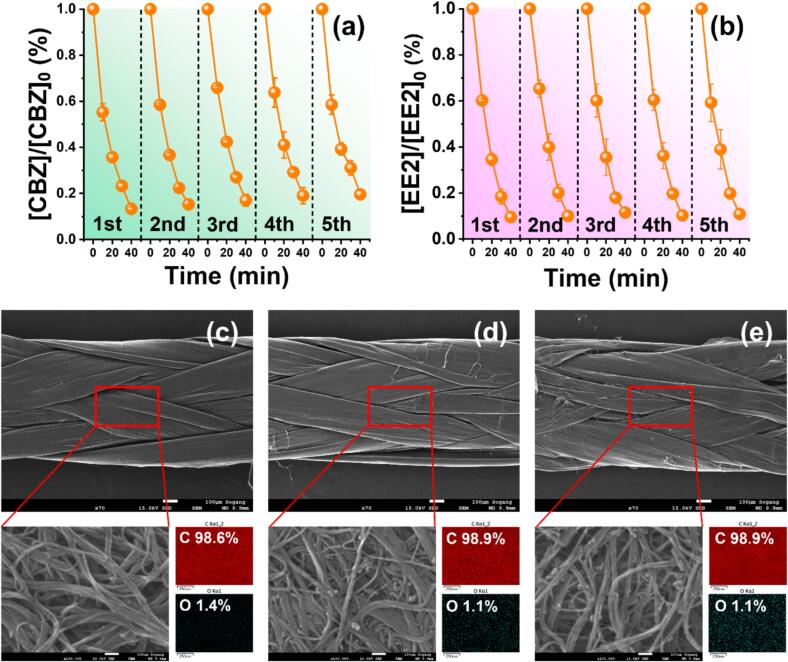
Reusability of CNTy over five consecutive treatment cycles for (a) CBZ and (b) EE2 ([CBZ]_0_ = [EE2]_0_ = 10.0 μM, pH 7.0, 100 W/L, [CNTy] = 200 mg/L, 5 mm, 40 min, 300 rpm, and 295 K). SEM images and EDS mapping of CNTy surfaces: (c) pristine CNTy, (d) CNTy after 1 h ultrasonic treatment, and (e) CNTy after five consecutive cycles.
